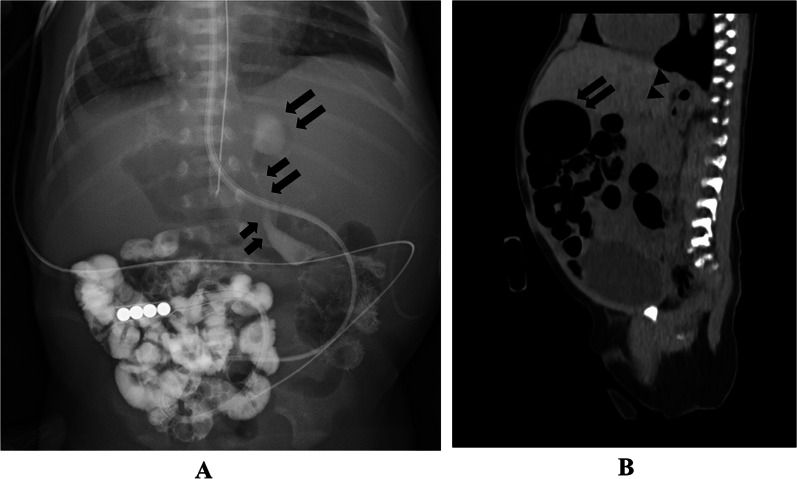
Postoperative imaging studies. A Postoperative gastrointestinal film showed a contrast agent pathway from the oral side of the side-to-side anastomosis to the dorsal area of the stomach, which was considered as remaining duplication (arrow). A 5-Fr tube was placed near the distal area of the anastomosis. B An abdominal computed tomogram revealed a luminal structure on the stomach’s dorsal area, which was compatible with the duplicated alimentary tract (arrow head). The stomach is located under the liver (arrow)
